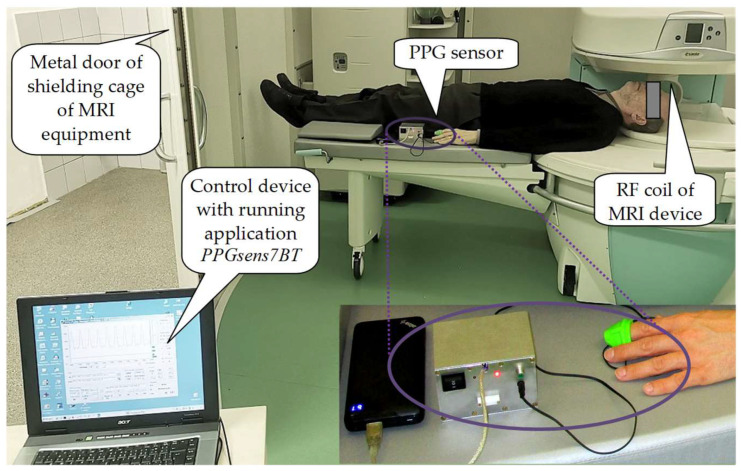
Measurement of a person lying in the MRI device Opera using the *PPG-EP* sensor with BT data transmission to a control device located outside the shielding metal cage.
